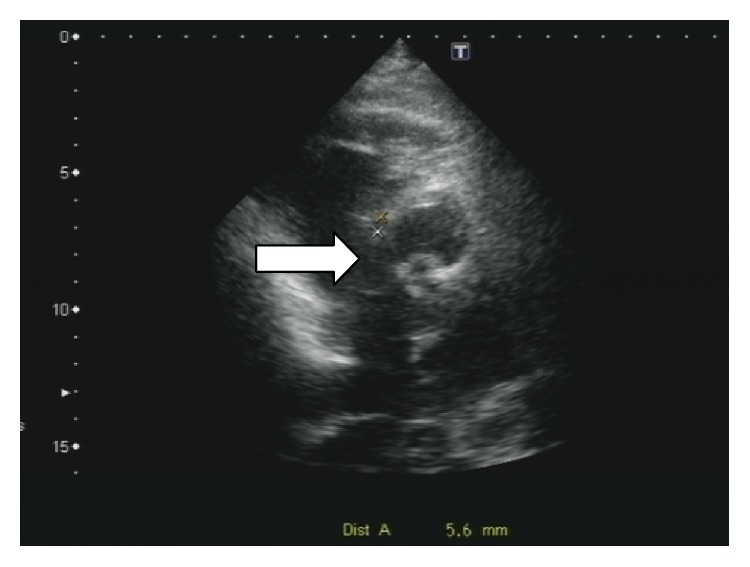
Ventricular septal defect (VSD). Parasternal short view showed the VSD (arrow).
